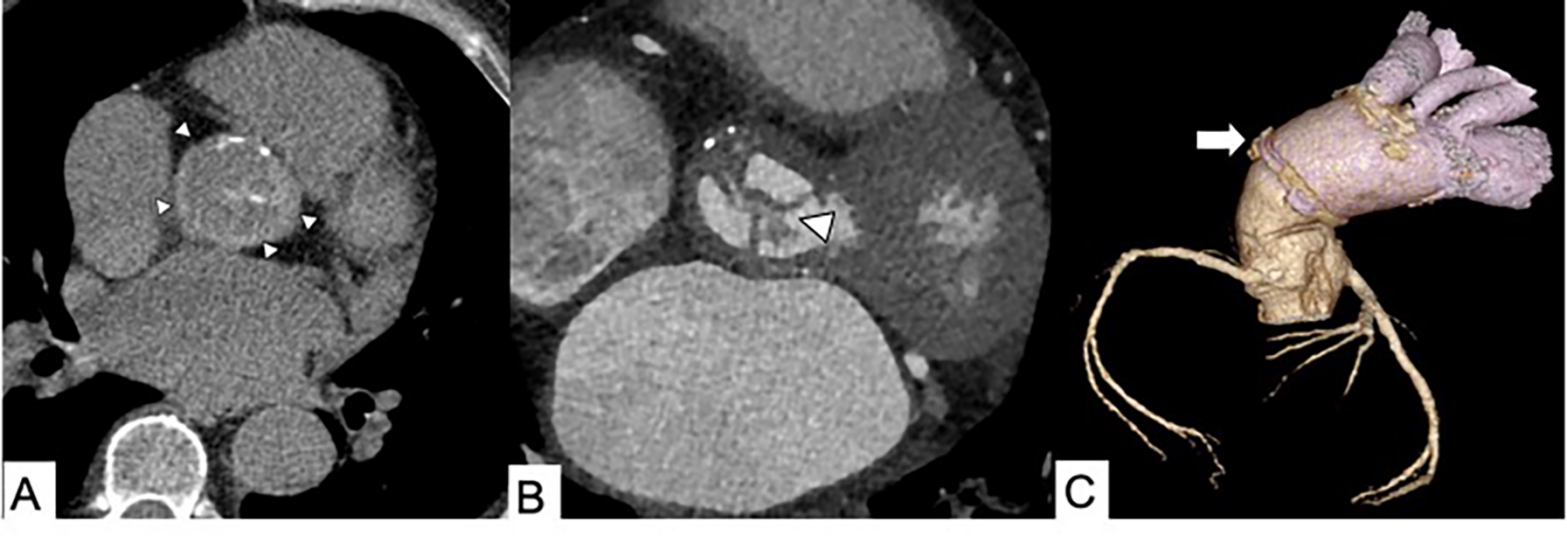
Infected endocarditis in a 65-year-old male patient with aortic bioprosthetic valved conduit. (A) A non-enhanced axial CT scan shows a hyperattenuating aortic prosthetic wall (arrowheads). (B) Thickening of prosthetic valvular leaflets with endocarditis vegetation (arrowhead) and (C) a 3D volume rendering reconstruction showing distal graft aortic anastomosis (arrow).
